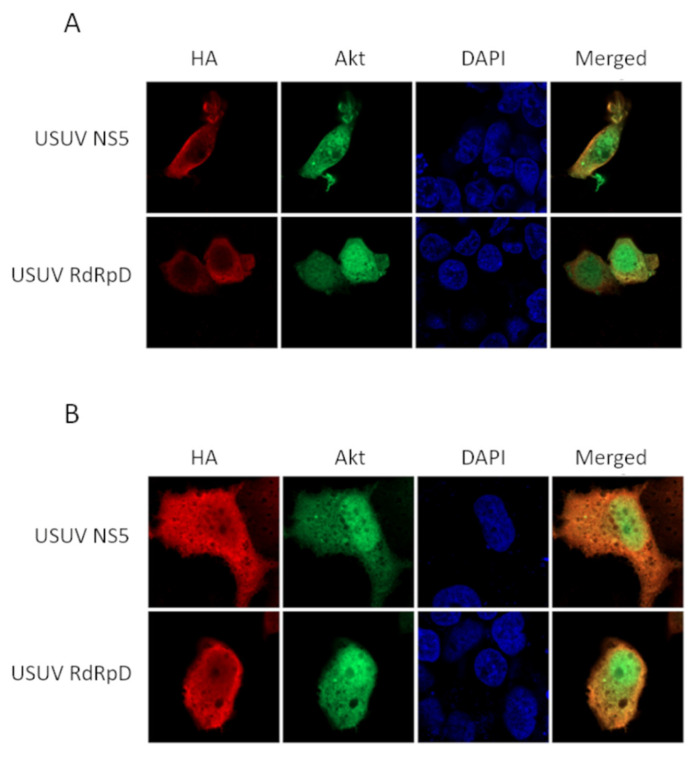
Intracellular localization of USUV NS5 or RdRpD, and Akt. (A) HEK-293T cells were transiently transfected adding two plasmids; one encoding Akt (pcLN-Akt-GFP) and another with the USUV protein (pcDNA3-USUV_NS5 or pcDNA3-USUV_RdRpD). The immunocytochemistry experiments were performed using antibodies against HA, showed in red, detecting USUV NS5 and USUV RdRpD. DAPI (in blue) was used to detect cell nuclei. Akt is expressed as a GFP-fusion protein, and hence a green signal associated with this protein is detected. On the right panel, we can see the merge of red and green channel images. Merged images show an overlay with co-localization in orange. (B) Same experiment with Huh-7.5 cells.
